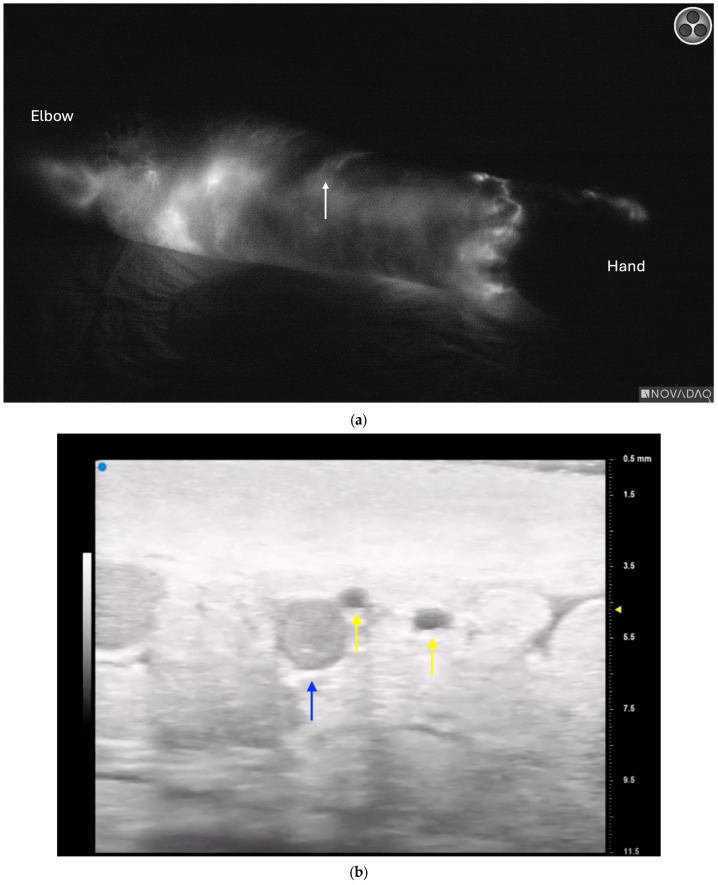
Ultra-high frequency ultrasound can identify lymphatic channels in more advanced lymphedema patients. (a) ICG lymphography of upper extremity demonstrating diffuse dermal backflow with splash pattern distally. Dilated lymphatic channels identified within backflow (*white arrow*) using UHFUS (b), which demonstrated two lymphatic channels (*yellow arrows*) and nearby venule (*blue arrow*). Patient of senior author A.A.S.
